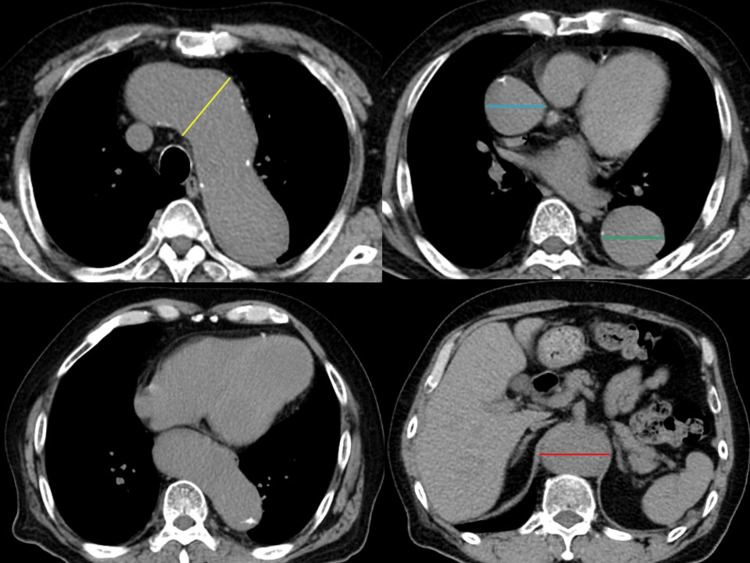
Preoperative plain computed tomography (CT) shows an extensive aortic aneurysm with a thoracoabdominal aneurysm CT showed that the ascending arch, descending aorta, and thoracoabdominal aorta measured 46 mm (blue line), 53 mm (yellow line), 43 mm (green line), and 55 mm (red line), respectively.
